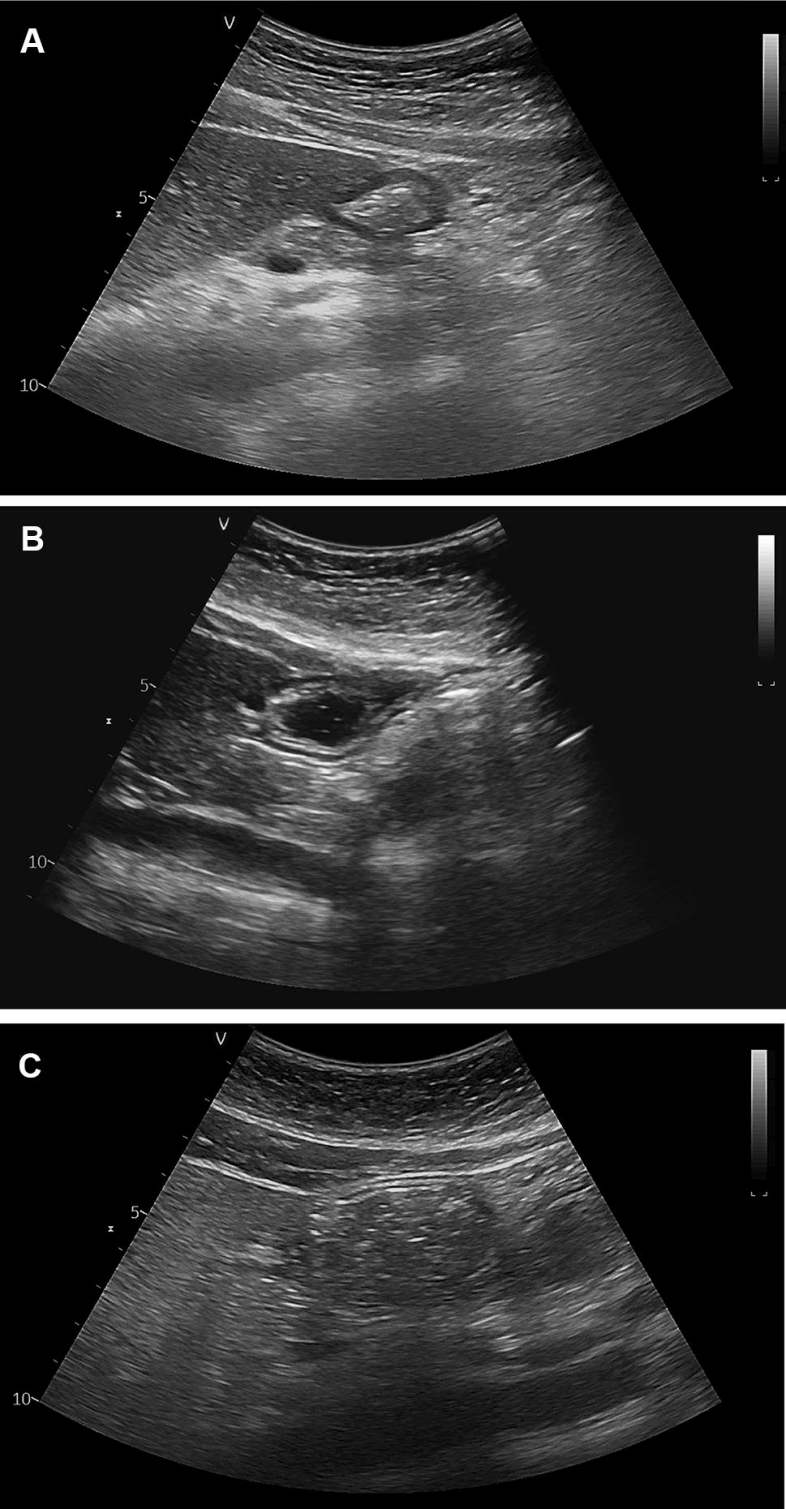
Ultrasound image of the gastric antrum in the epigastric area obtained in a sagittal or parasagittal plane according to gastric contents. The empty antrum (grade 0) is presented (A) in the right lateral decubitus, The antrum with minimal, insignificant amount of fluid (grade 1) appears (B) in the right lateral decubitus; The antrum with significant fluid content (grade 2) is detectable (C) in the right lateral decubitus.
